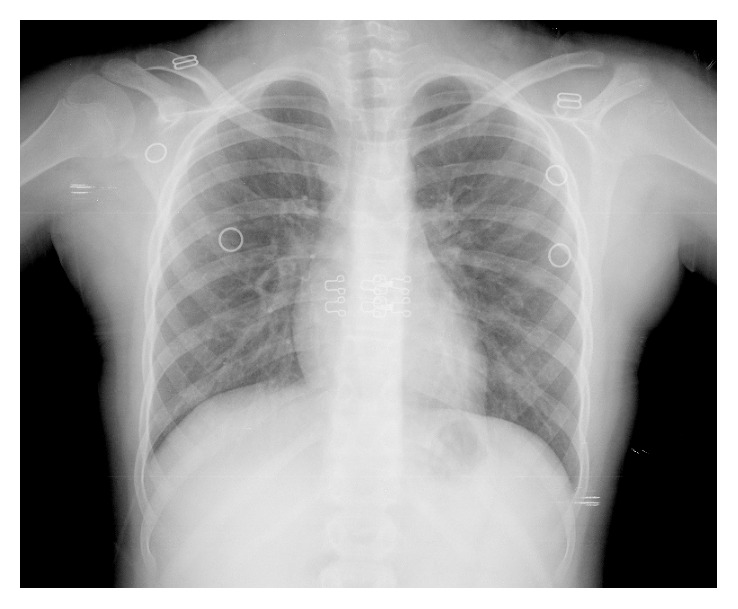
Chest radiograph showed the steeple sign.
